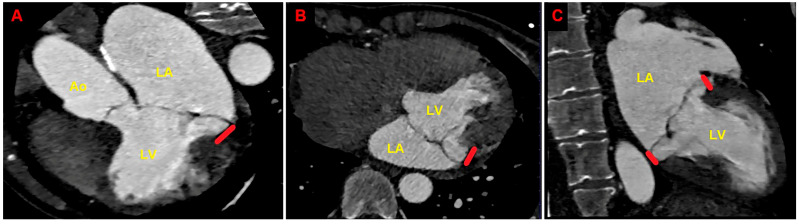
Cardiac computed tomography angiography. MAD assessment at end-systole from the multiplanar reconstructed three-chamber view (A), four-chamber view (B), and two-chamber view (C). The bold red line indicates the MAD distance. Ao, aorta; LA; left atrium; LV, left ventricle. (A) is reproduced from the ref. [4].
